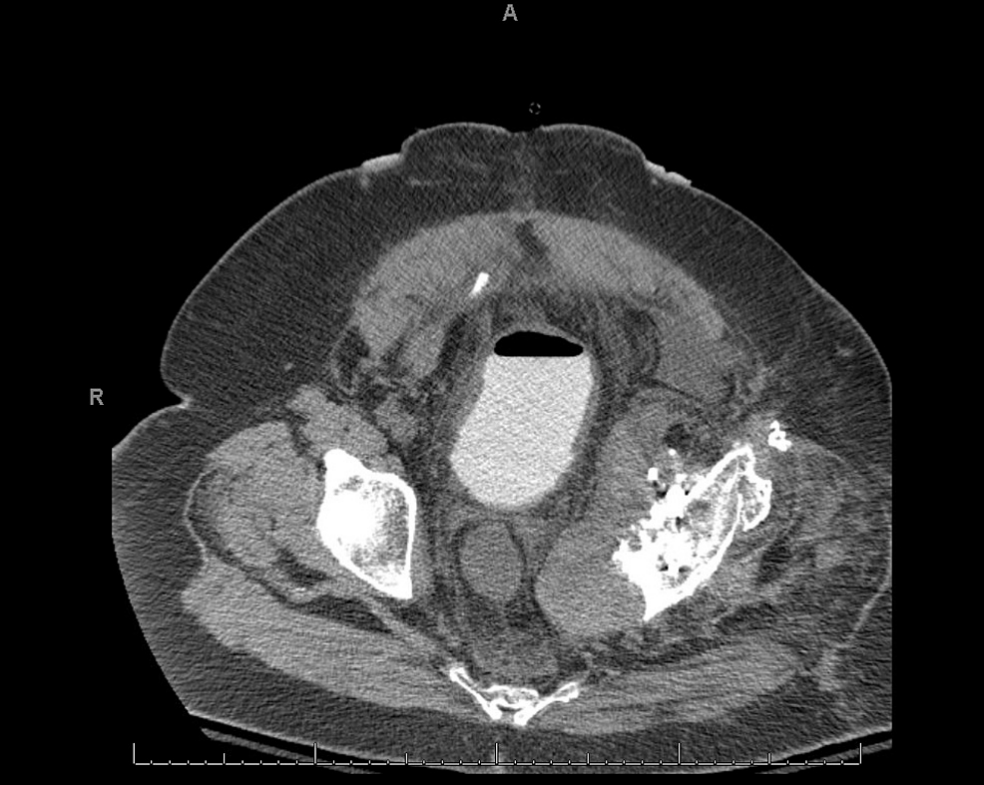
Postoperative CT scan. Postoperative axial CT scan demonstrating decompression of pseudotumor, with now normal-appearing location of the bladder and rectum without narrowing as seen preoperatively.
